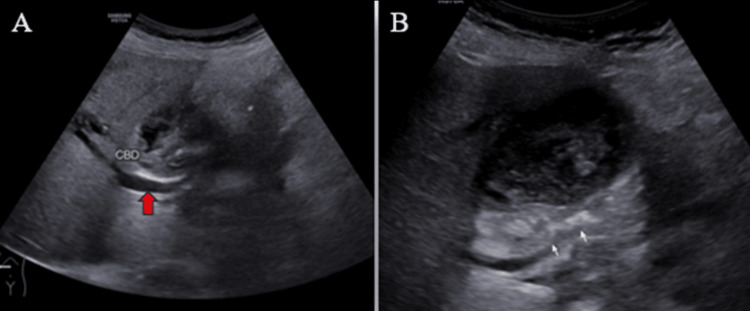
Ultrasonography images show the dilated proximal CBD (red arrow) (A) and a heterogeneously hypoechoic lesion (white arrow) in the mid-CBD, accompanied by narrowing of this part of the CBD (B). CBD: Common bile duct
